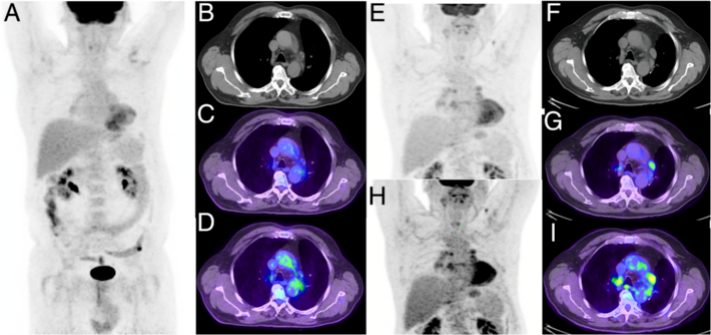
Patient with non-small cell lung cancer treated with curative intent radiotherapy. Post treatment restaging PET/CT demonstrated a complete metabolic response (a–d, c upper SUV threshold adjusted to liver background as detailed above, d upper SUV threshold of 5). Follow-up CT 9 months later demonstrated enlargement of multiple mediastinal nodes considered likely to represent malignant aetiology. Repeat PET/CT (e–i) demonstrated low-to-moderate uptake in these nodes. Given the symmetry of distribution in hilar and mediastinal nodes the aetiology was considered inflammatory, which was confirmed by resolution on follow-up. Thresholding the PET with a SUV threshold of 5 (h–i) might lead to erroneous description of intense uptake and interpretation as malignant in aetiology
